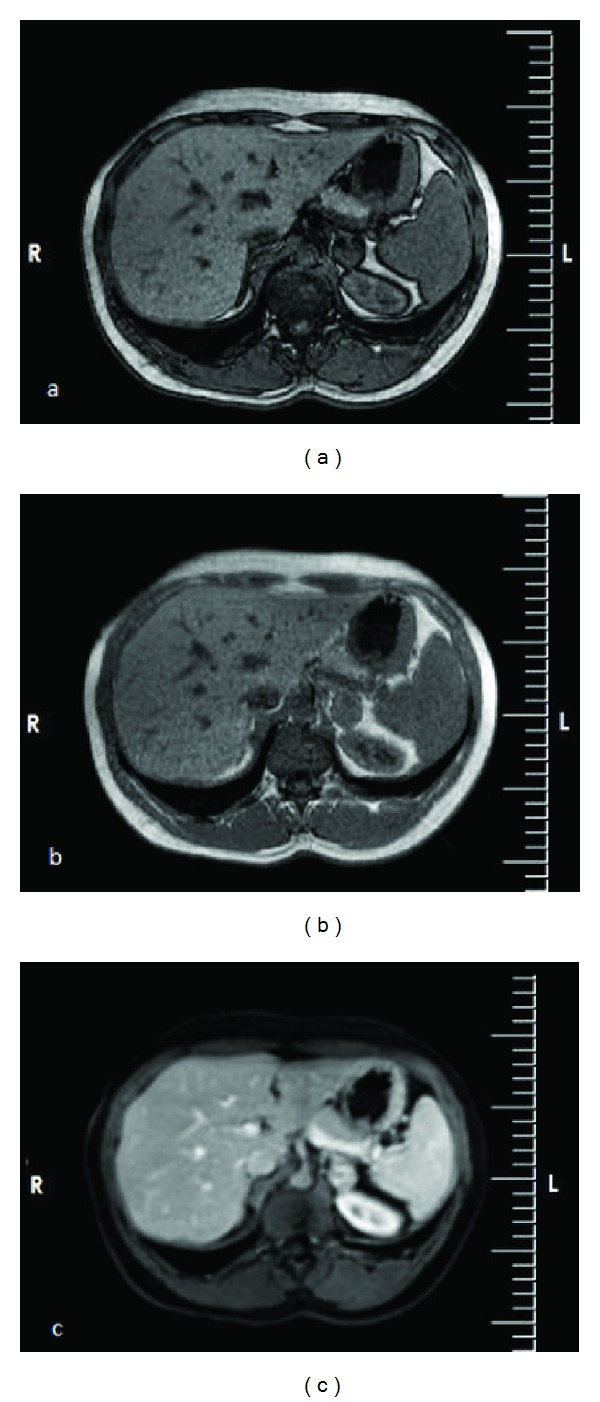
(a–c) Axial T1-weighted out-phase MR images (a), in-phase MR image (b), and three-dimensional GRE contrast-enhanced MR image show the intense mass in left adrenal gland. (c) shows no loss of signal intensity on the out-of-phase image.
